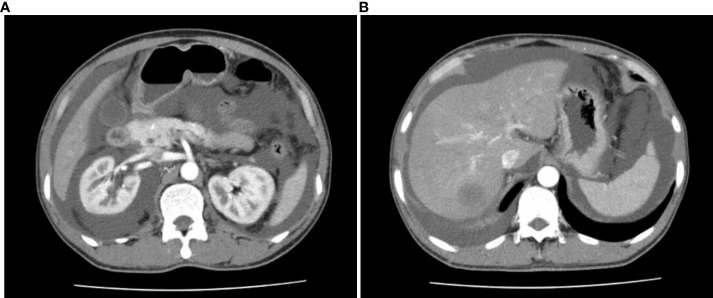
Enhanced CT scans of patient 2. (A) Perinephric fluids; (B) Abdominal fluids.
